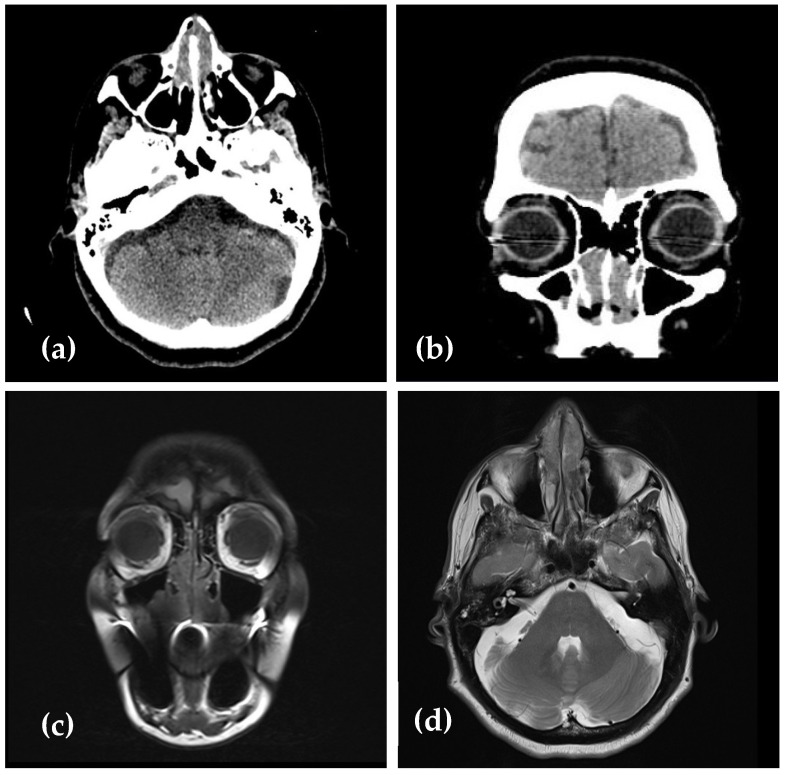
(a,b) Computed tomography scans of the head in the coronal and axial planes showing multiple, homogeneous, hypo-intense polypoid masses, which narrowed the nasal passages more extensively on the left side, obstructing the left choanal arch, without sinus involvement or bone erosion. (c,d) Coronal and axial T1-weighted images showing iso-intense to slightly hypo-intense lesions compared to gray matter.
